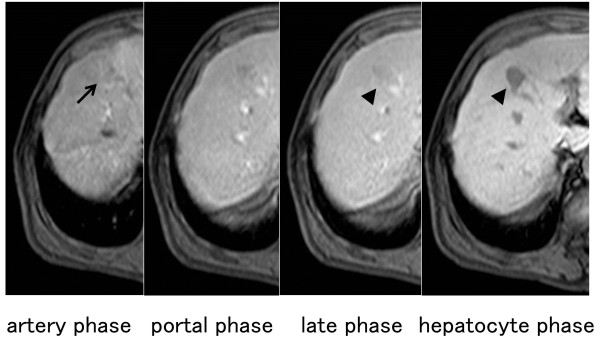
Dynamic contrast-enhanced magnetic resonance imaging (MRI) findings with gadolinium ethoxybenzyl diethylenetriamine pentaacetic acid (EOB-MRI). EOB-MRI showed that the tumor appears isointense in the arterial phase (arrow) and was completely washed out in the delayed and hepatocyte phases (arrow head).
